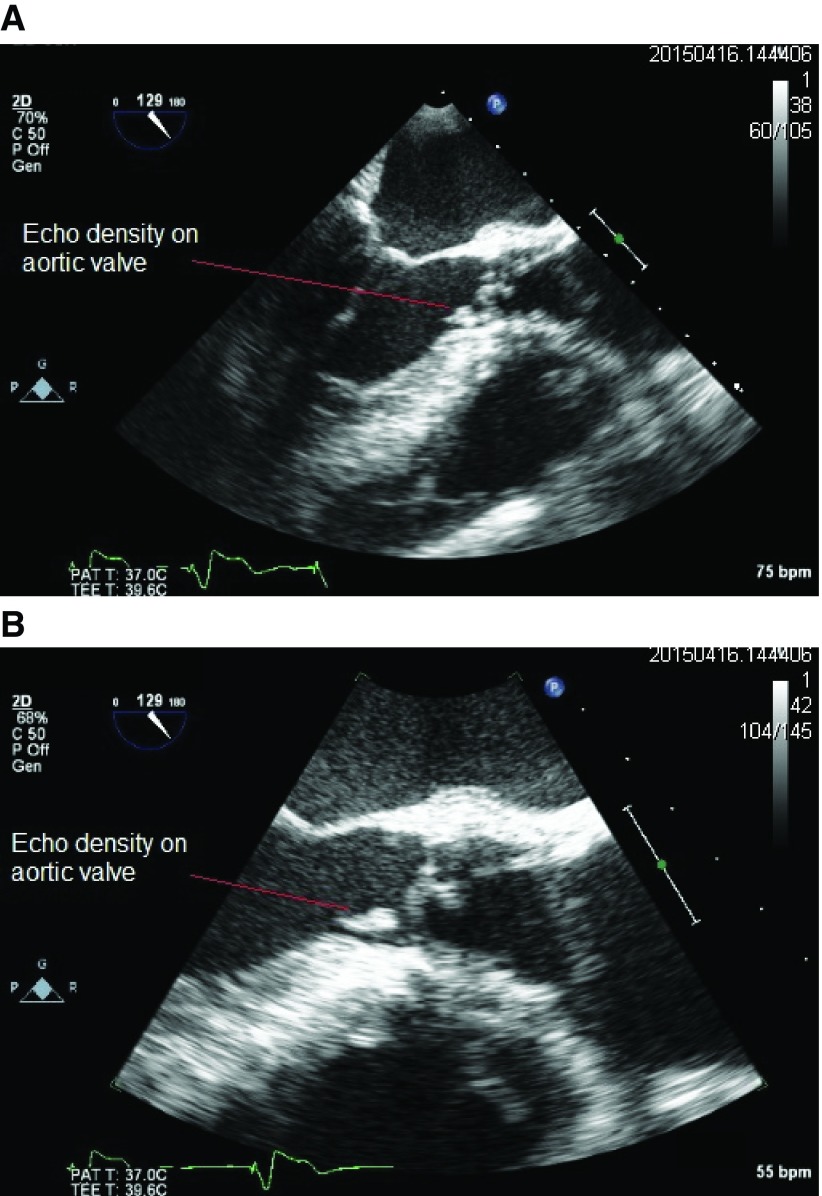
1A: Transesophageal echocardiogram (TEE), mid-esophageal view showing mobile echo density on the prosthetic aortic valve.
